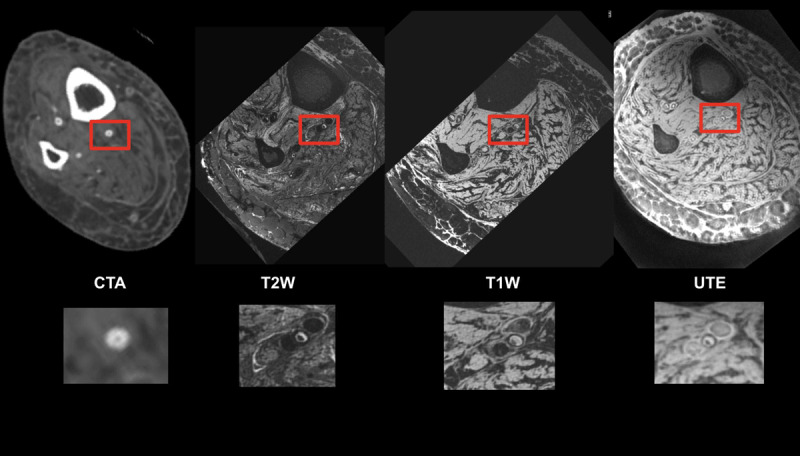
Tibial vessels imaged with computed tomography angiography (CTA) and magnetic resonance imaging (MRI) using T2-weighted, T1-weighted, and ultrashort echo time sequences. The CTA shows concentric calcium but suffers from beam hardening artifacts and calcium blooming that obscures the vessel lumen. MRI with ultrashort echo time imaging shows a very thin rim of hypointensity around the central vessel, indicating that the calcium ring is thin and the lumen is occluded with mixed morphology plaque.^11^
